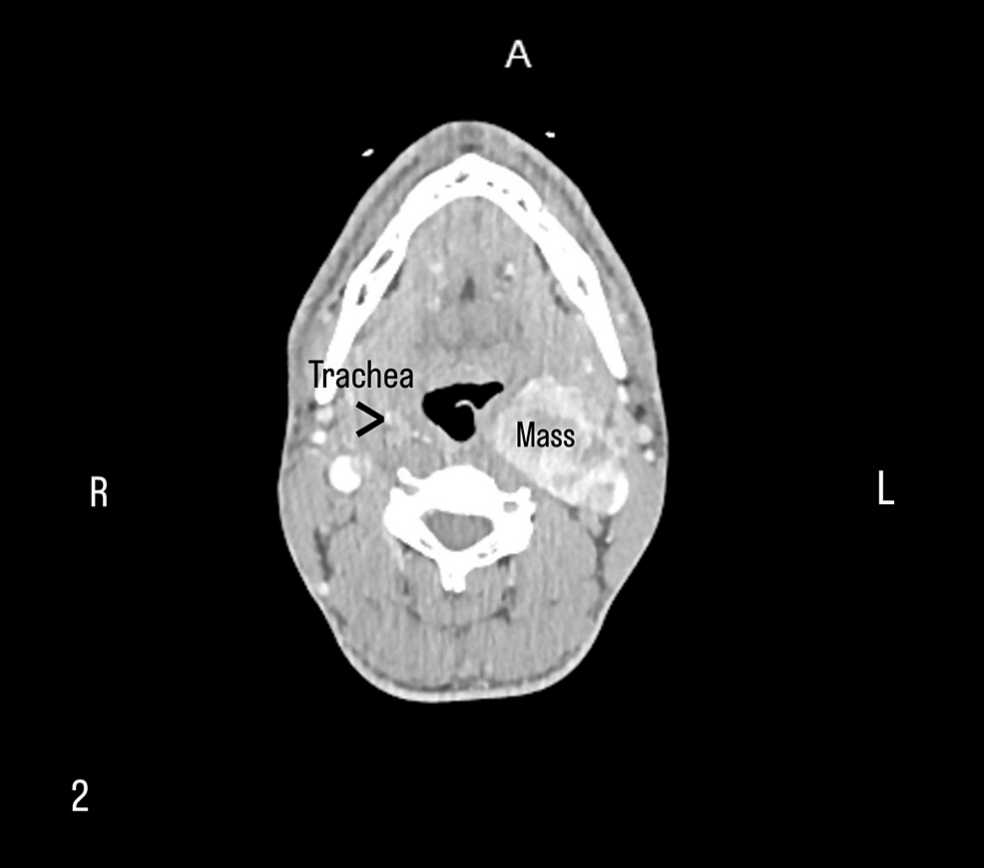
Computed tomography angiography of the neck shows partial compression of the trachea by the tumor.
